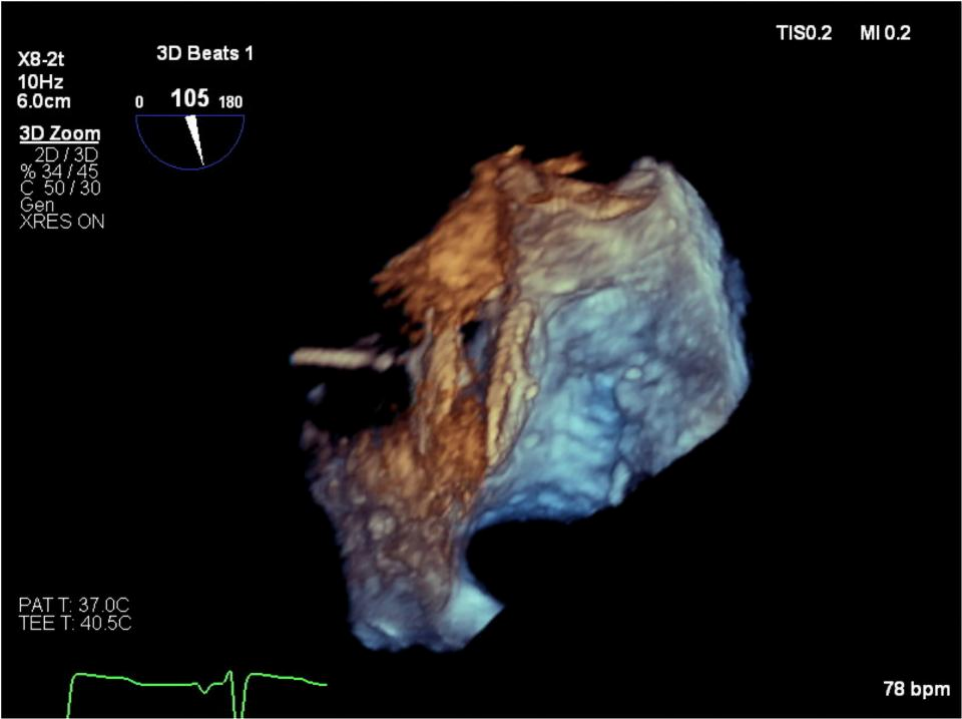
3D transoesophageal image of the patent foramen ovale occluder device *in situ* before release. The atrial septum is captured between the 30 mm right atrial disc and the 25 mm left atrial disc of the device, thus stabilizing the atrial septal aneurysm, closing the foramen ovale and eliminating the right-to-left shunt.
